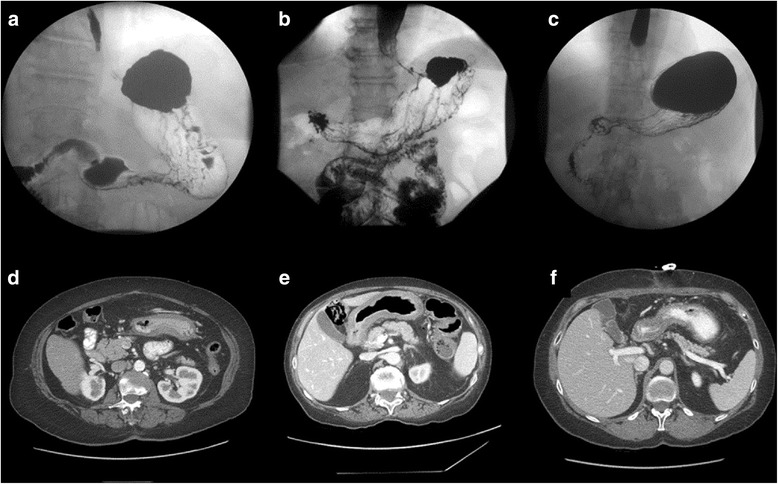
Upper gastrointestinal imaging (a–c) and computed tomography (d–f) diagnostic features of linitis plastica in three different patients (patient 1: a, d; patient 2: b, e; patient 3: c, f)
